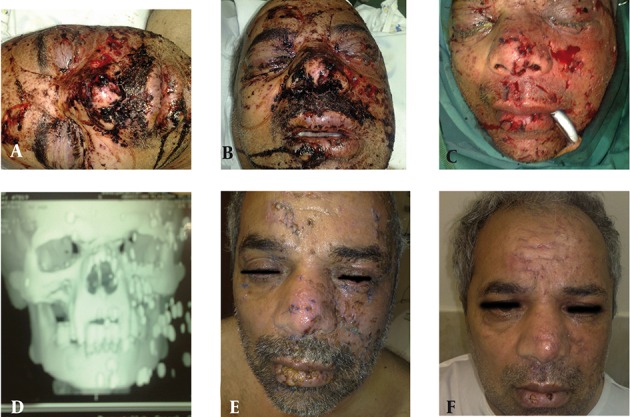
A 50 year-old man with burn-blast traumatic tattoo, a,b-before operation, c-intra operative view, d-x-ray view, e-early post operation, f-three months postoperation.
